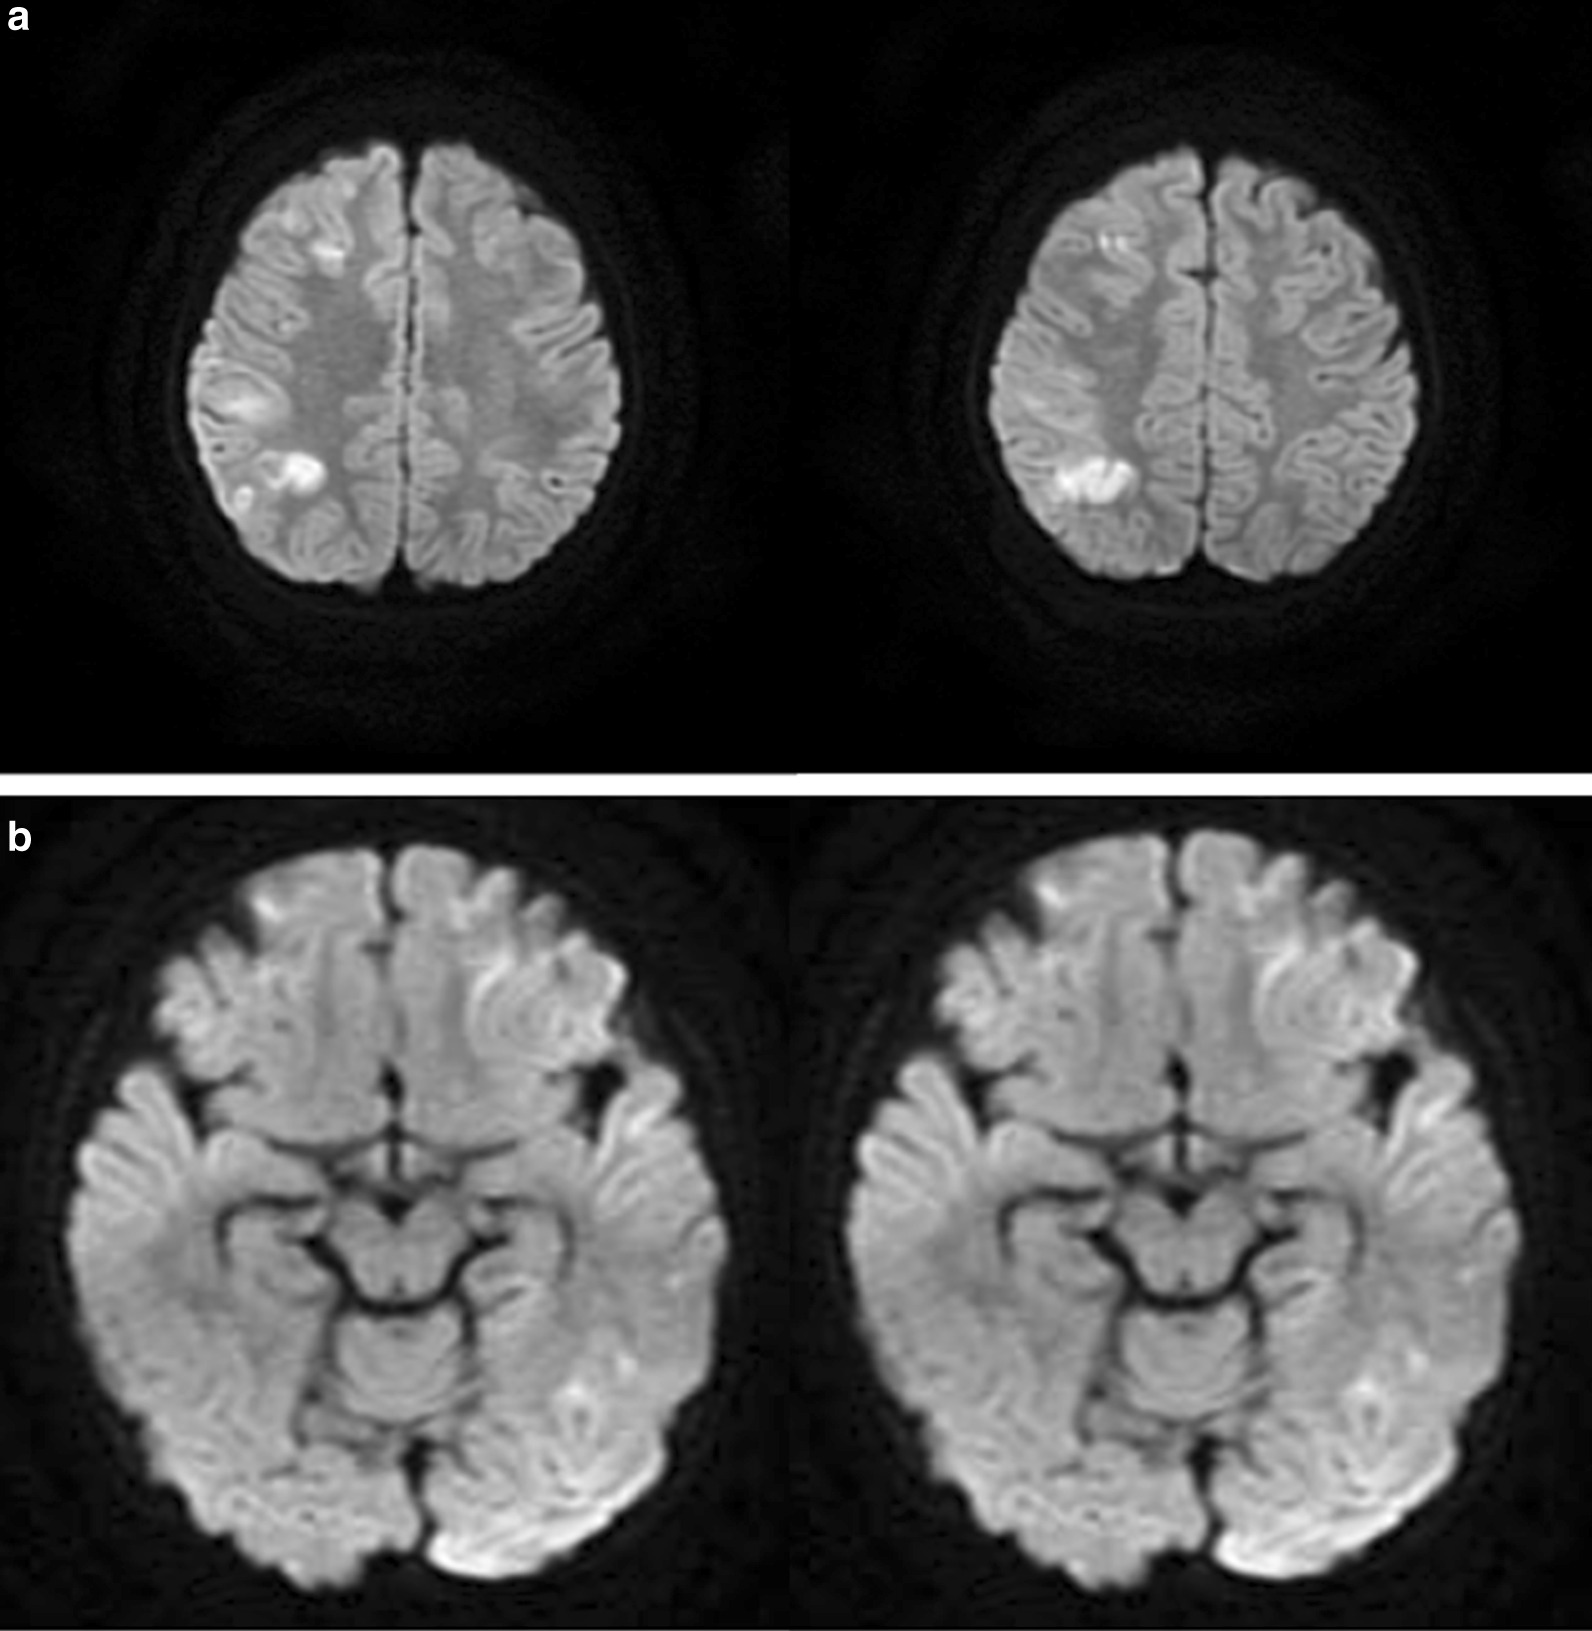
Brain diffusion-weighted magnetic resonance imaging (MRI-DWI) in case 1 (a) and case 2 (b). a Brain MRI findings (axial; diffusion-weighted images) of case 1 (first hemiplegic episode). Cortical restricted diffusion signals were seen predominantly in the right frontal and parietal lobe during the episode of left hemiplegia. b Brain MRI findings (axial; diffusion-weighted images) of case 2. Mild hyperintensity was seen in the left hemispheric cortex during the episode of right hemiplegia
